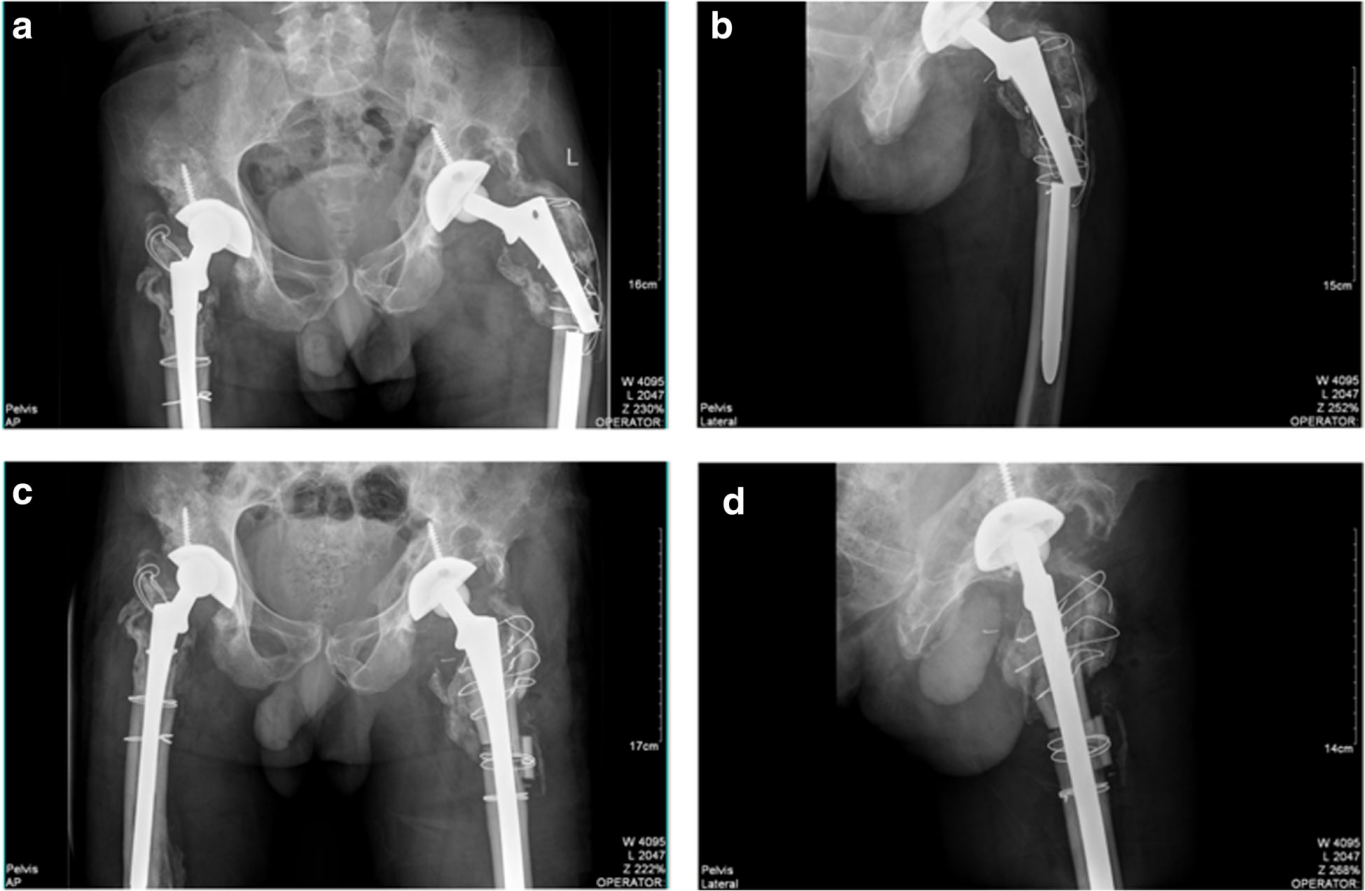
a and b: Pre-operative AP radiographs taken in December 2014 showing the left femoral stem fracture. c and d: Radiographs taken after revision of the left hip
